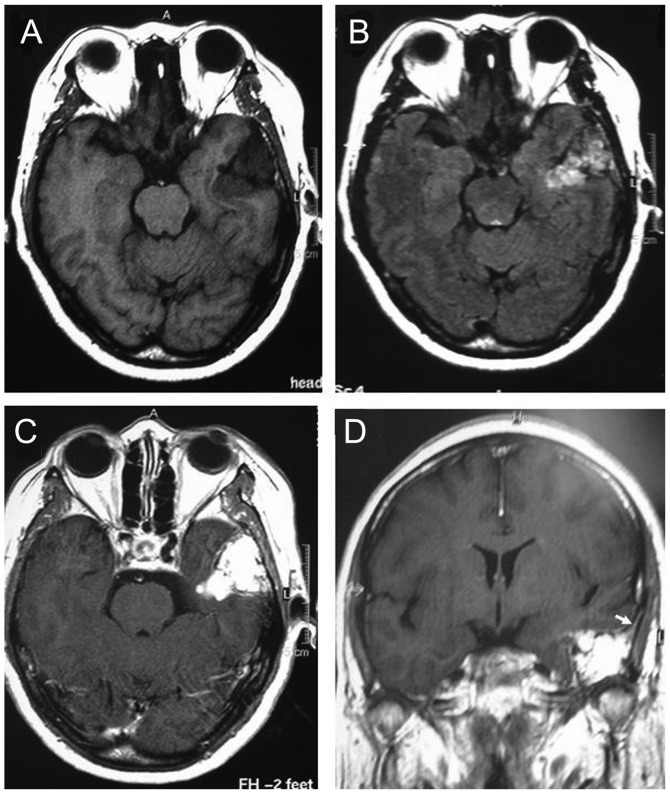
Magnetic resonance imaging prior to surgery. (A) Axial T1-weighted MRI revealing a hypointense lesion in the right petrous bone. (B) Axial T2-weighted magnetic resonance imaging showing the lesion to be hyperintense. (C) Axial and (D) coronal gadolinium-enhanced T1-weighted magnetic resonance imaging showing a heterogeneously enhanced lesion, with a dural tail sign (arrows).
